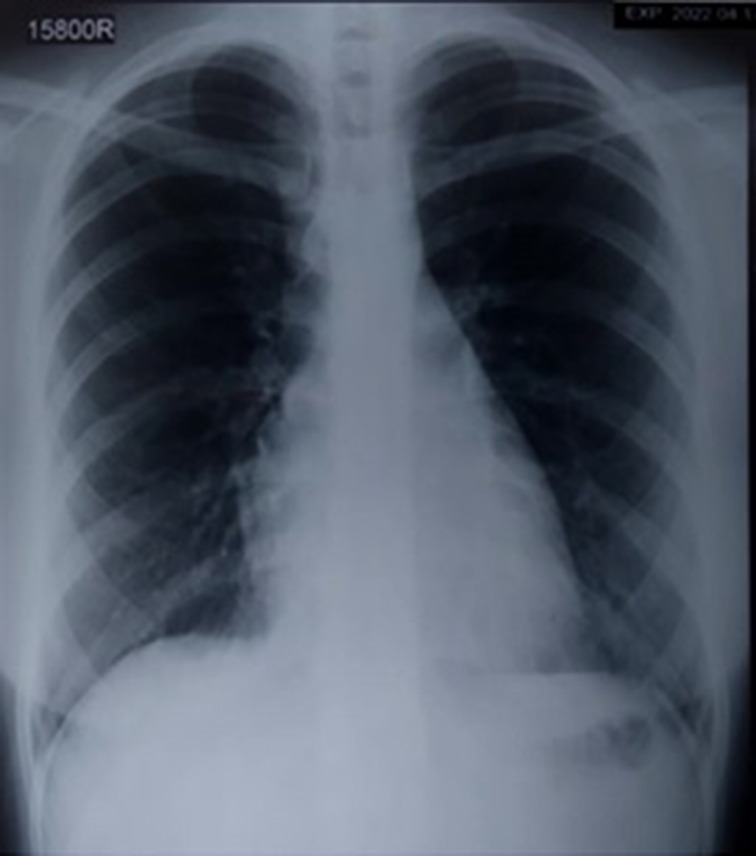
Chest radiograph after 1 month of recovery.
